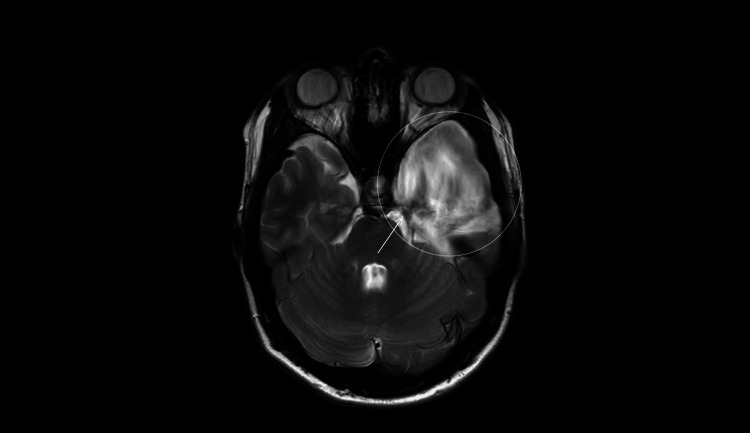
MRI (March 5, 2025; axial T2-weighted; 4-mm slices; 0.5-mm gap). Flow-related signal heterogeneity within the left middle-fossa arachnoid cyst (open circle) along its medial wall (arrow) makes the cyst-basal cistern communication less conspicuous on T2 at this time point.
